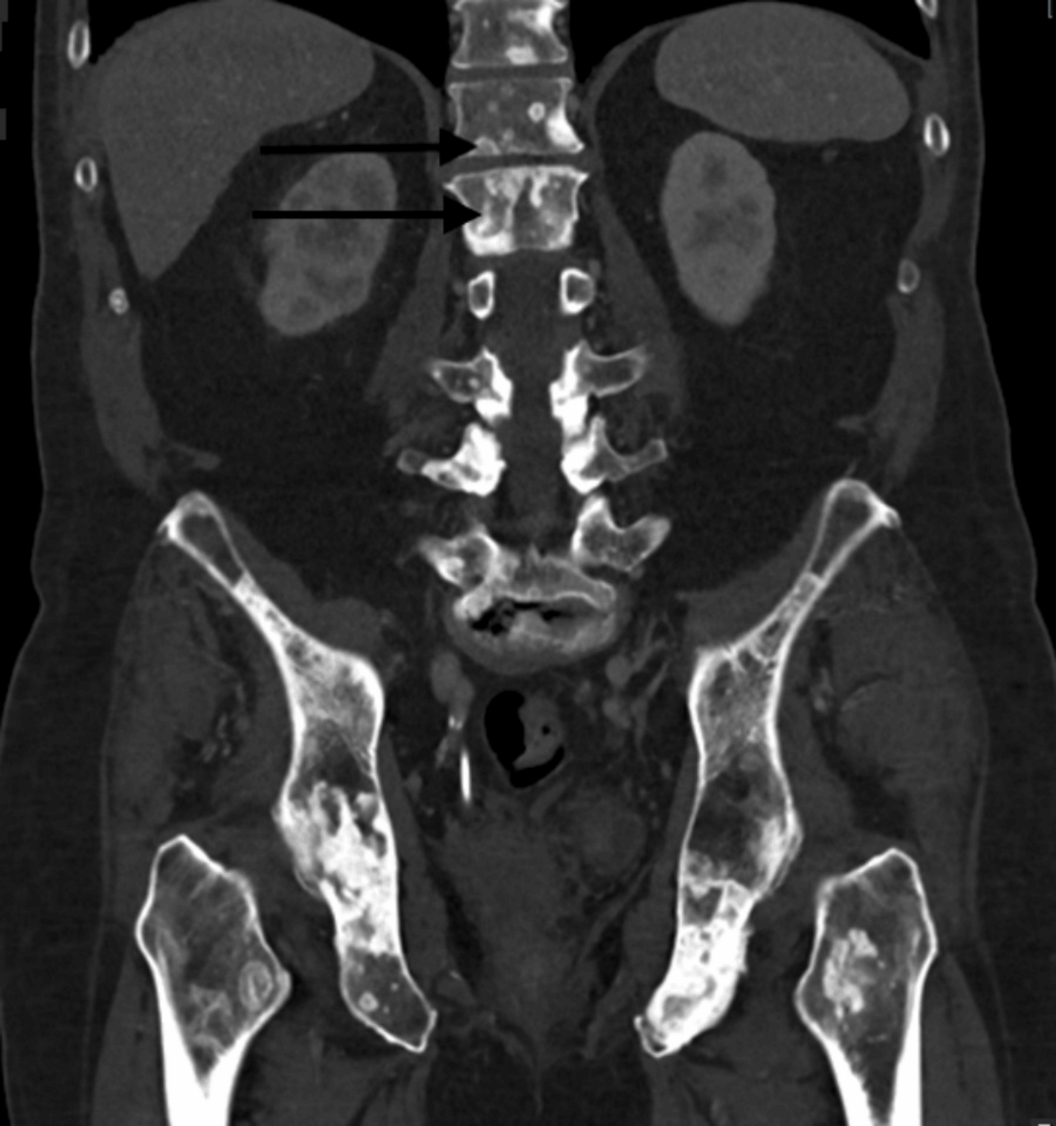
CT showing multiple osseous metastases in the coronal view
